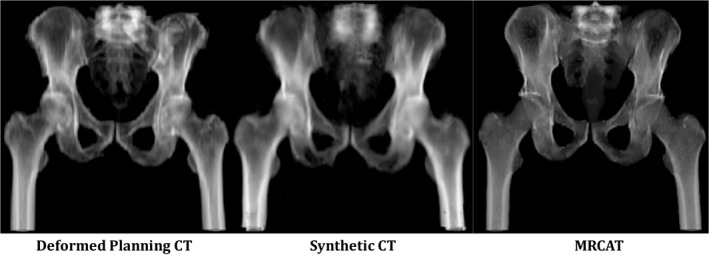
Example of digitally reconstructed radiograph (DRR) images corresponding to deformed planning CT (Left), our proposed Synthetic CT (Middle) and MRCAT CT (Right). The lower quality of the DRR in the superior region of the synthetic CT is mainly due to lack of data in our atlas in those regions. DVH summary and dose distribution for this patient are shown in Figs. 5 and 6 respectively.
